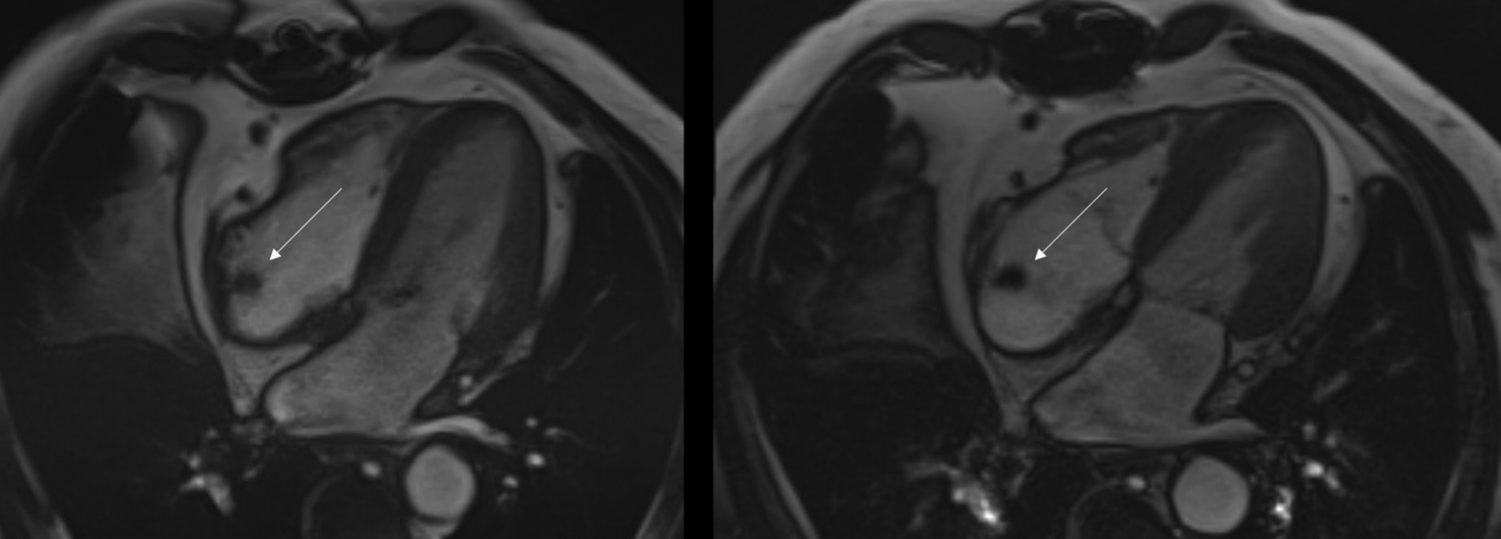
Figure 2: Cardiac MRI without contrast. Further, evaluation with MRI cardiac morphology was performed. The study was limited due to motion and refusal to administer contrast. TRUFI (true fast imaging with steady-state free precession) axial images in four-chamber view demonstrates a prominent right atrial intracavitary lesion
